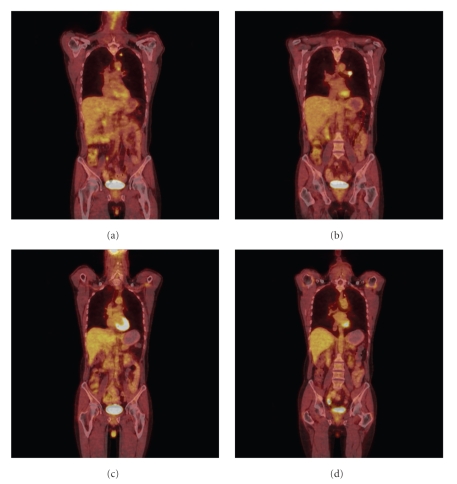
Coronal views of FDG-PET/CT scan obtained before (a)-(b) and after (c)-(d) treatment for primary NSCLC in patient with synchronous SBM. At diagnosis, the patient demonstrated hypermetabolic activity in a primary LUL tumor with mediastinal (a) and hilar (b)lymphadenopathy. Two years after treatment, including surgical resection, chemotherapy, and mediastinal RT, there is no evidence of hypermetabolic activity at the prior sites of thoracic involvement (c)-(d).
